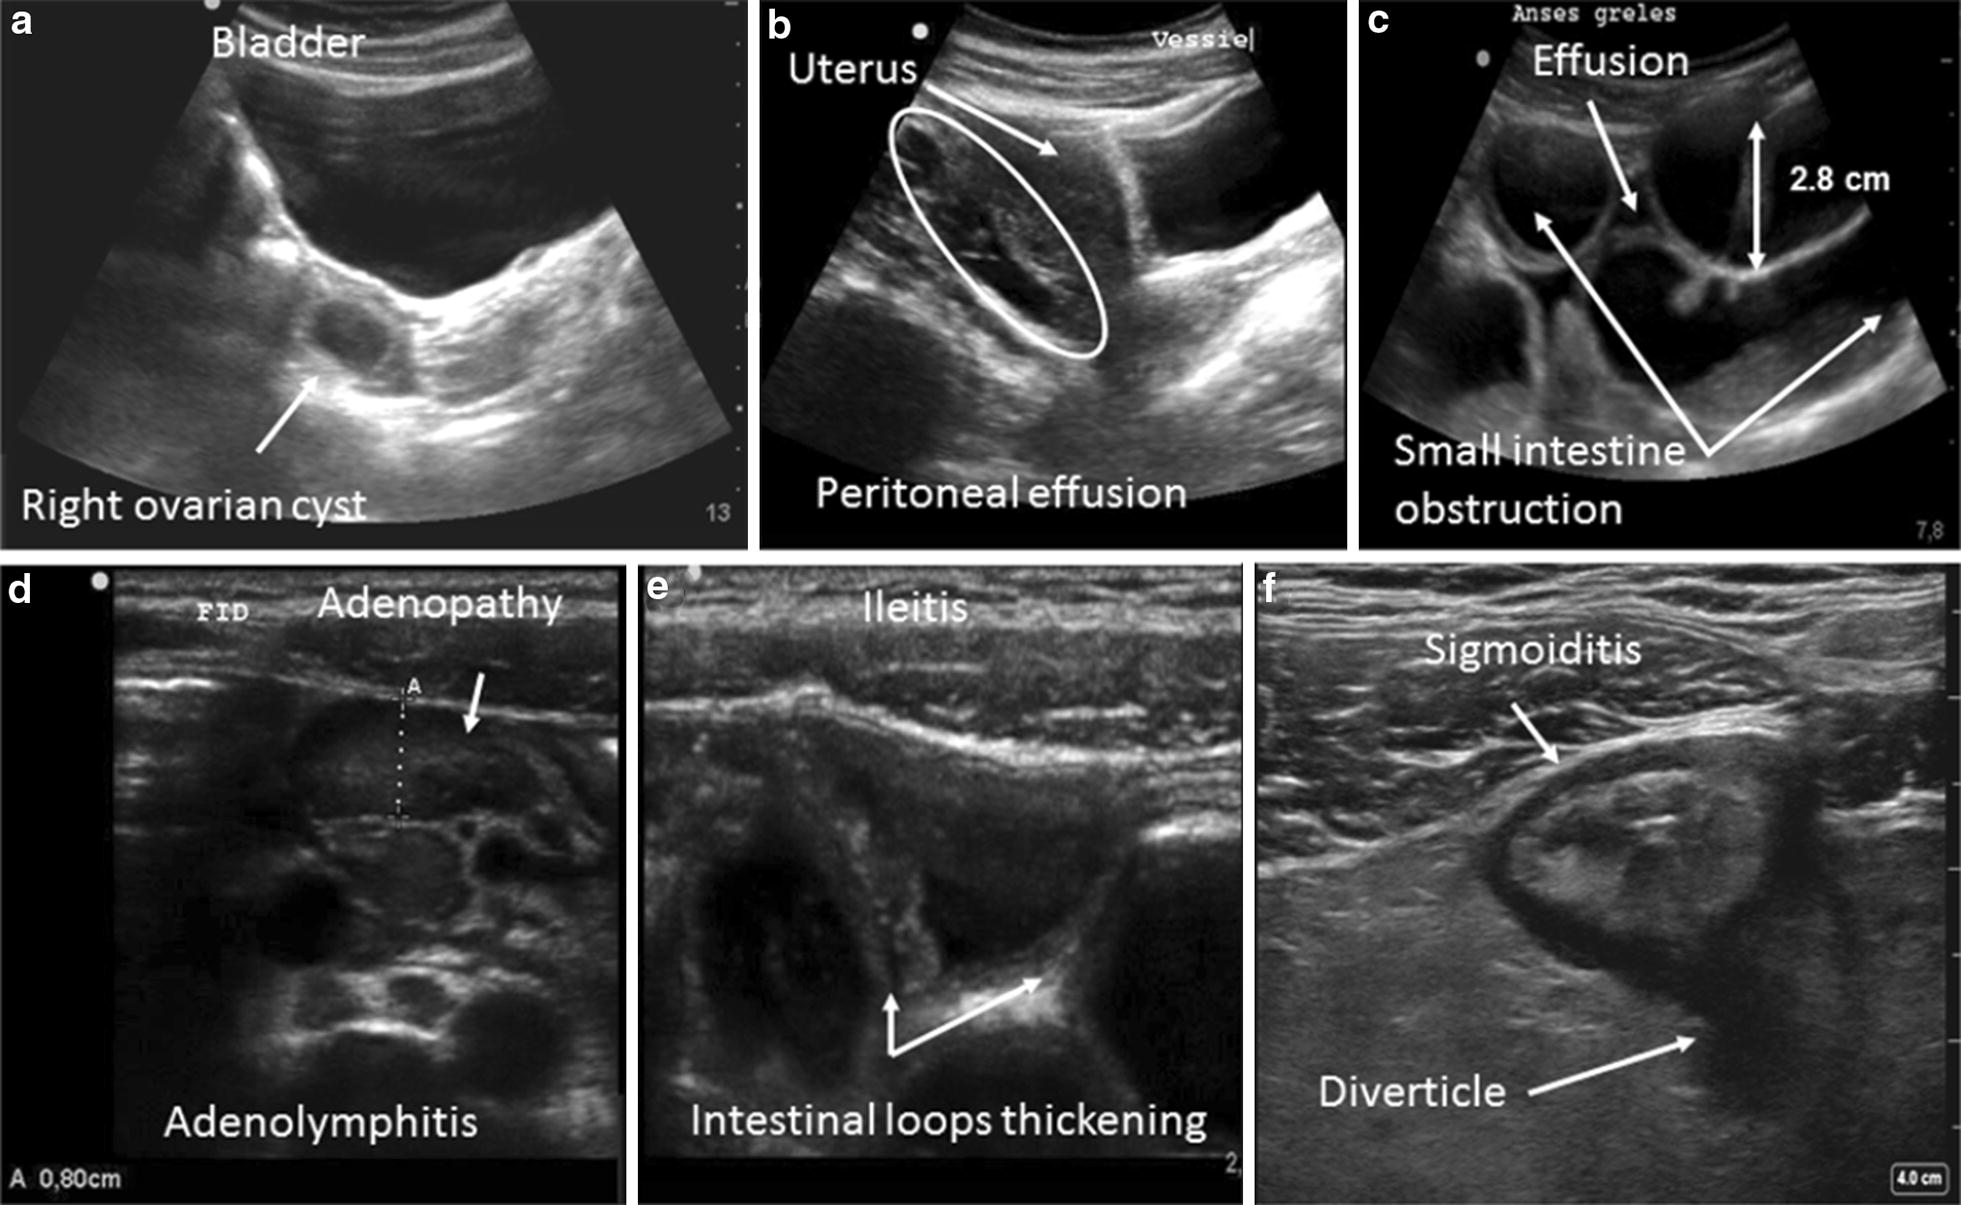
Examples of abnormal ultrasound images: a a latero-uterine mass with a sonolucent liquid content suggestive of a complicated ovarian cyst, b isolated peritoneal effusion (often at the bottom of the Douglas pouch), c appearance of a small intestinal occlusion (widening of the intestinal loops by more than 2.5 cm, with a liquid content and possible inter-loop effusion), d adenopathies (hypoechoic oval images greater than 5 mm in terms of the antero-posterior diameter, sensitive to passage of the probe, and enhanced by color Doppler), e thickening of the last small intestinal loops suggestive of ileitis (thickening by more than 3 mm of the wall of the digestive tract), f circumferential widening of the colon wall (a pseudo-kidney appearance) suggestive of an inflammation or an infection
